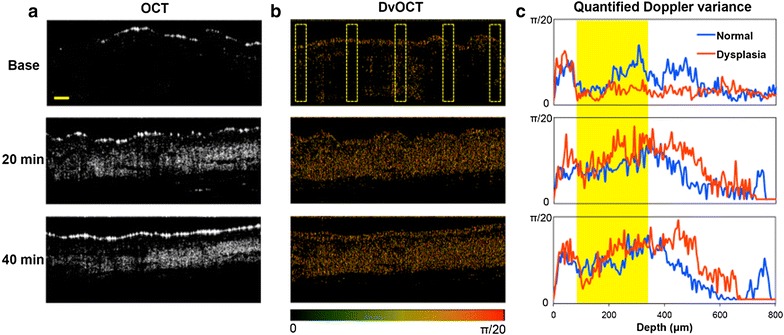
OCT and DvOCT imaging of dysplastic hamster check pouch with acid-disassembling Au NCs. Images of DMBA-applied, early-stage dysplastic hamster cheek pouch, obtained by SD-OCT (a) and DvOCT (b) after administering acid-disassembling Au NCs. Scale bar: 100 µm. The images were taken before (base) and 20 and 40 min after administering acid-disassembling Au NCs. The color-coded Doppler variance in DvOCT images of early-stage dysplasia (5 dotted regions) was quantified and compared with that of normal tissue (c) (yellow area: epithelial layers)
